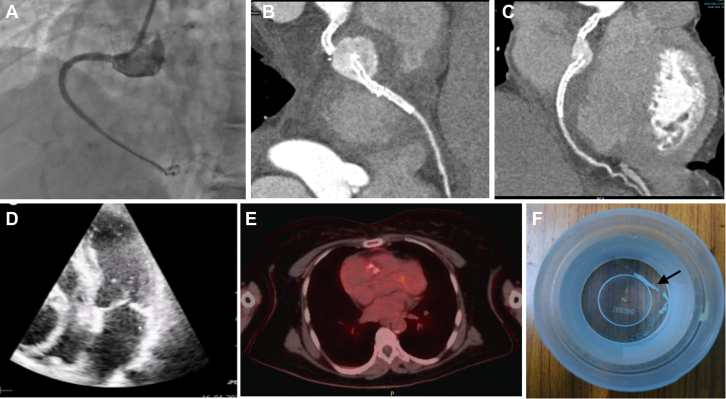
Case 4: Right Coronary Artery–Infected Pseudoaneurysm With Negative Positron Emission Tomography-Computed Tomography Result and Surgical Retrieval (A) Final angiographic result after index percutaneous coronary intervention at right coronary artery with TIMI flow grade 3. (B and C) Coronary computed tomography angiography after fever onset demonstrating pseudoaneurysm involving the stented right coronary artery segment with stent fracture. (D) Apical 4-chamber echocardiographic view showing a large pseudoaneurysm anterior to the right atrium. (E) Fluorodeoxyglucose positron emission tomography-computed tomography showing no significant uptake at the pseudoaneurysm site. (F) Retrieved stent material from the pseudoaneurysm cavity (black arrow).
